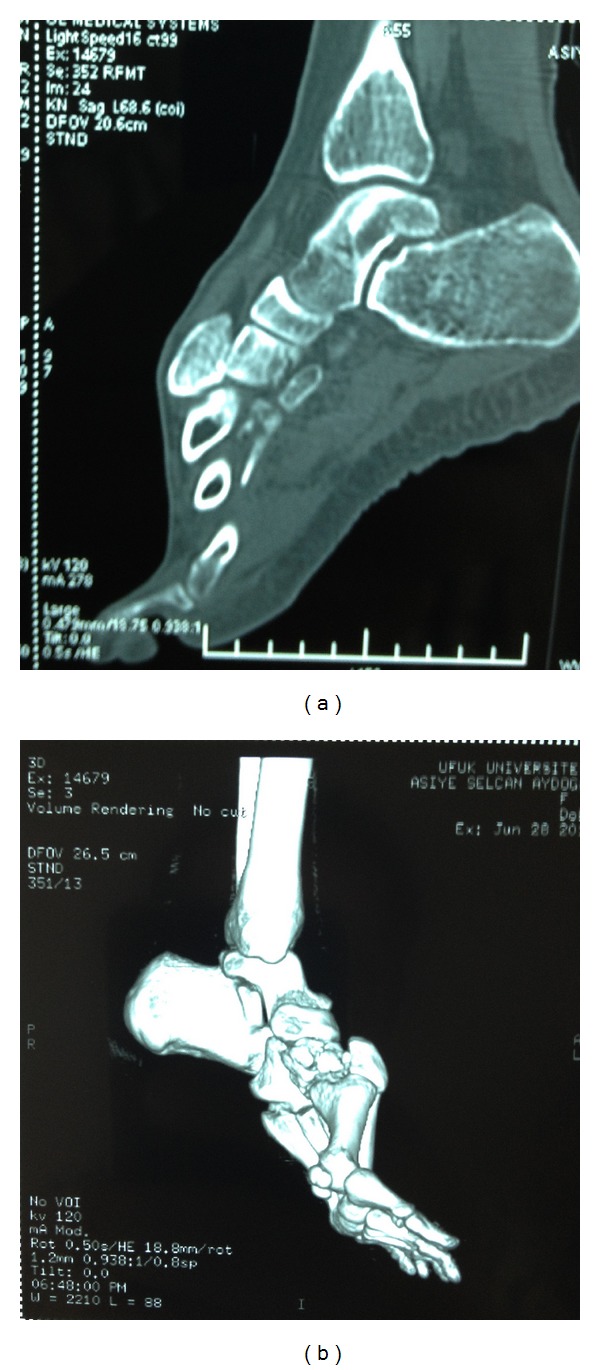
(a) Preoperative sagittal scan of computed tomography. (b) Preoperative three-dimensional reconstruction of computed tomography.
